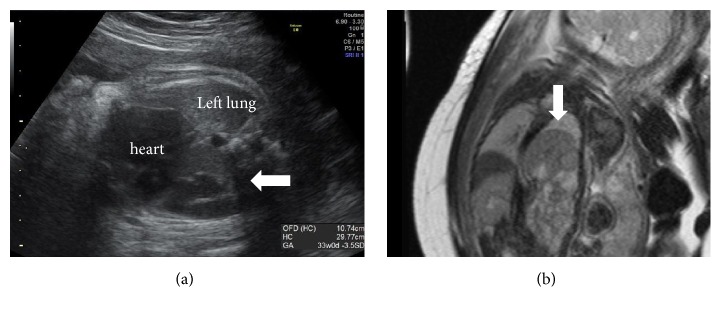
(a) Fetal echography showed a smooth-surface cystic lesion 29 × 44 mm in diameter in the left dorsal thorax* (arrow)*. (b) Magnetic resonance imaging at 37 weeks of gestation suggested left sac-type CDH. The stomach and spleen were herniated into the left chest* (arrow)*.
